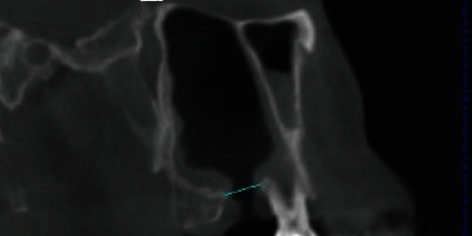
Cone beam computed tomography (CBTC) showing the oroantral communication in sagittal view.
